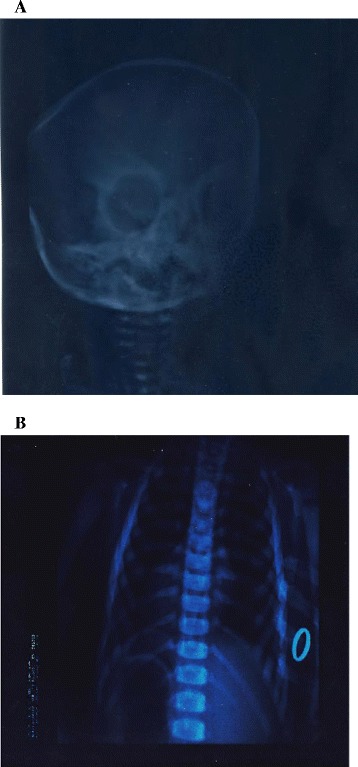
Radiographic analysis of a case with CLCN7 dependent OP. A) Skull view showing thickening of the inner and outer cortical tables. B) Chest showing generalised osteosclerosis in the thoracic cage.
